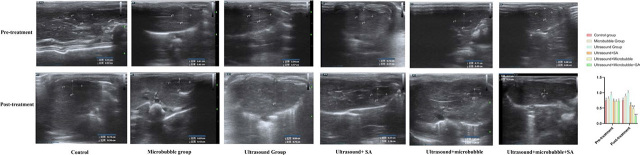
The changes in tumor size before and after drug treatment detected by Ultrasound images (n = 6; ^*^Compared with Pre-treatment group, *P* < 0.05; ^^Compared with Pre-treatment group, *P* < 0.01).
